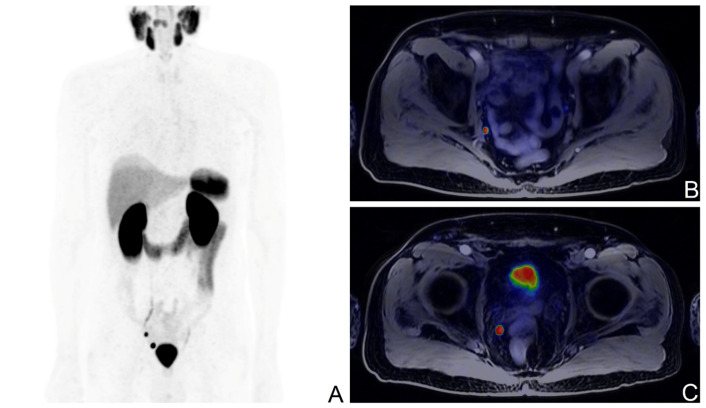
[^68^Ga]Ga-PSMA-11 PET/MR scan of a BCR patient with the ISUP score of 3, pT3b after RP; PSA levels at the time of the scan: 0.14 ng/mL; Maximum Intensity Projection image (A), fused axial PET/MR views showing a pathological right iliac lymph node (B), and a pathological right pararectal lymph node (C).
